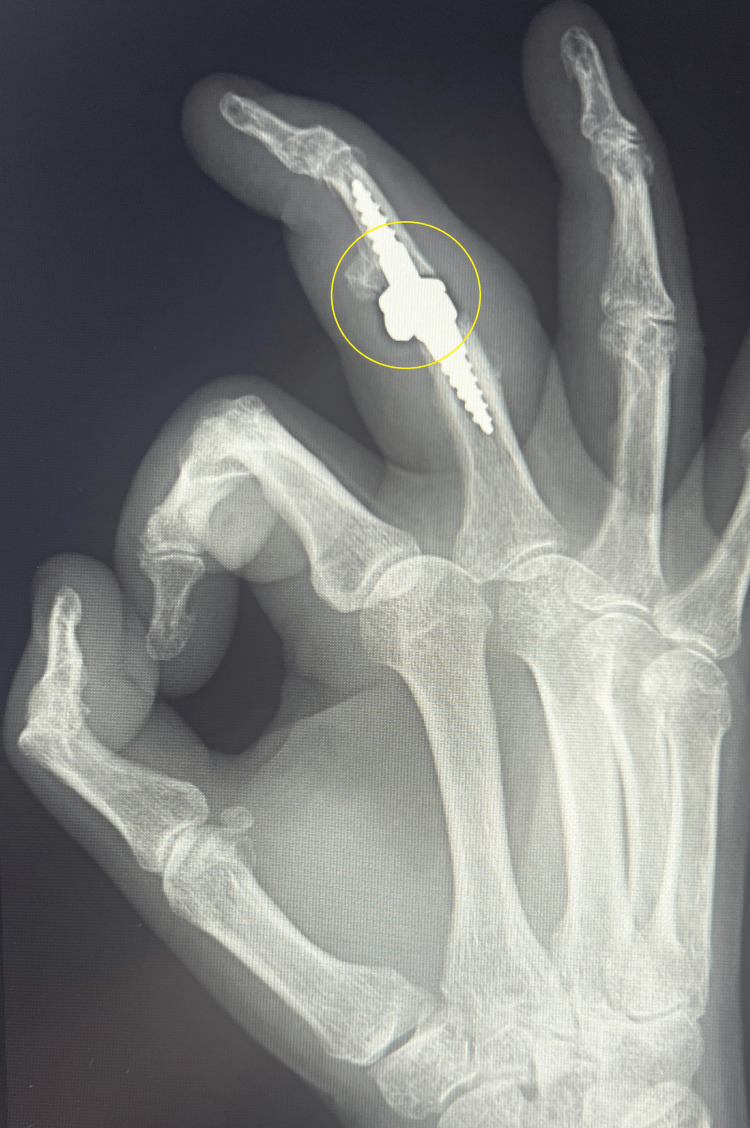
Control X-ray in Robert's projection obtained after surgery
